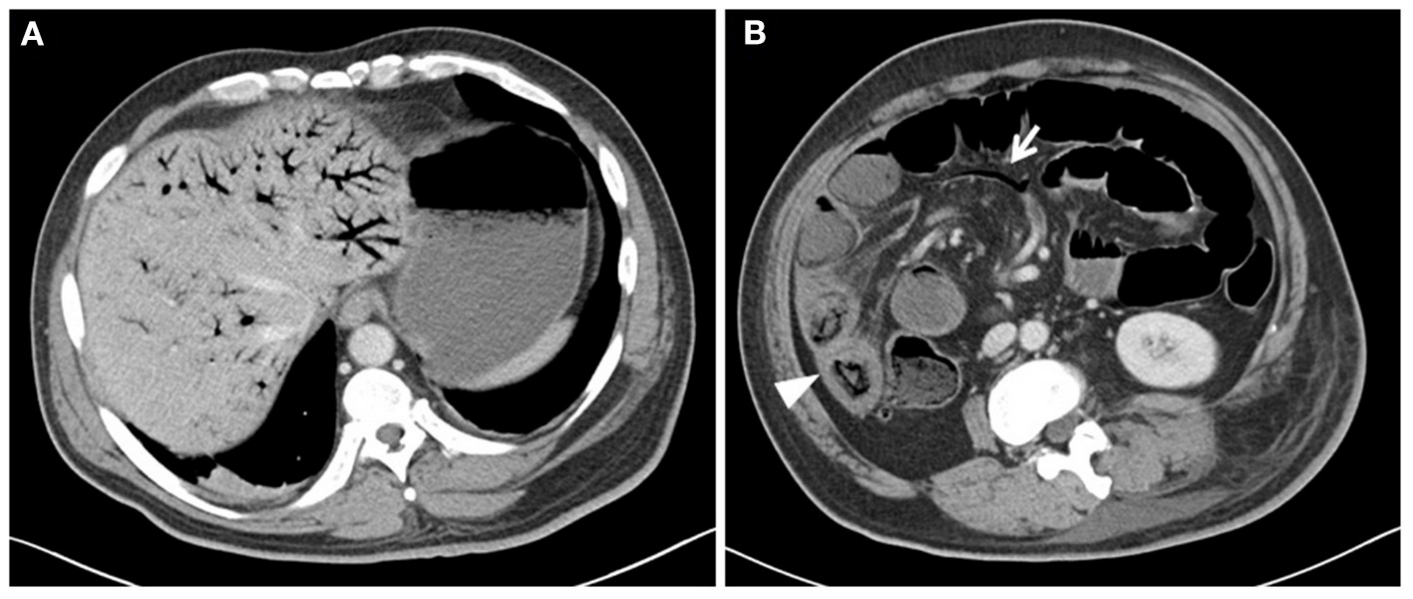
Abdominal computed tomography (CT) showed extensive portal (A), mesenteric vessels gas [(B), arrow], and dilated bowel loops. One segment of swollen small intestine [(B), arrowhead] without obvious pneumatosis intestinalis was also observed in the CT scan.
